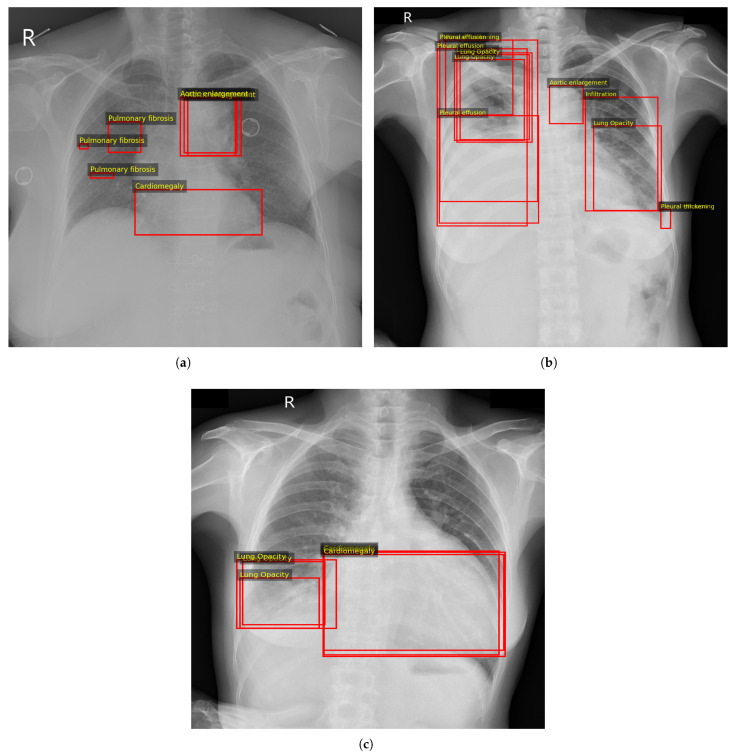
Examples of VinBigData radiologist bounding-box annotations overlaid with Grad-CAM visualisations for selected thoracic abnormalities. Red rectangular frames indicate expert-annotated regions of interest corresponding to abnormal findings. The letter “R” denotes the right side of the chest radiograph. Multi-label targets for the three examples are listed in Table 2. (a) Example 1. (b) Example 2. (c) Example 3.
